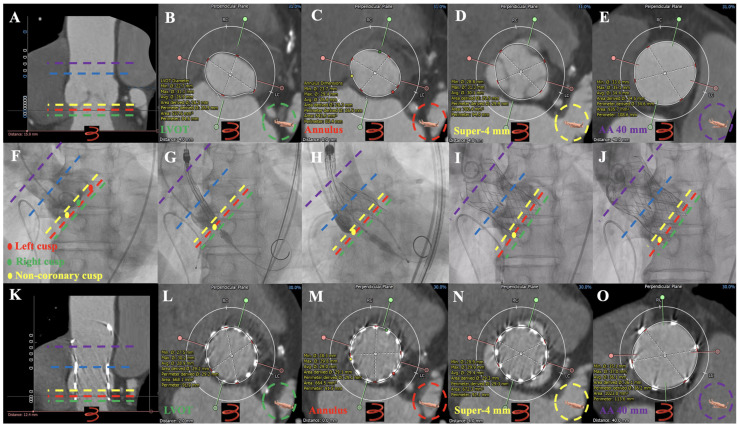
Dual anchoring measurements in Preoperative MDCT, Operative Fluro, Follow-up MDCT images in one case of severe AR underwent TAVR guided using MDCT anatomical classifications: type 1. Preoperative MDCT: (A–E): aortic root imaging at longitudinal section, LVOT, annulus, super-annular 4 mm, and AA 40 mm in patients with type 1. Operative Fluro: (F)*:* aortic root angiography; (G)*:* initial positioning of 30 mm VitaFlow valve; (H): half-releasing at 180 bpm (pacing lead in right ventricular); (I,J): VitaFlow valve deployed completely. Follow-up MDCT: (K–O): aortic root imaging at longitudinal section, LVOT, annulus, super-annular 4 mm, and AA 40 mm in patients with 30 mm VitaFlow valve implantation. ● = Left Coronary cusp; ● = Right Coronary cusp; ● = Non-coronary cusp. ▬ ▬ ▬ ▬ = Annulus; ▬ ▬ ▬ ▬ = LVOT; ▬ ▬ ▬ ▬ = super-annular 4 mm; ▬ ▬ ▬ ▬  = AA 40 mm; ▬ ▬ ▬ ▬ = sinotubular junction. AR = aortic regurgitation; TAVR = transcatheter aortic valve replacement; MDCT = multidetector computed tomography; AA = ascending aorta.
